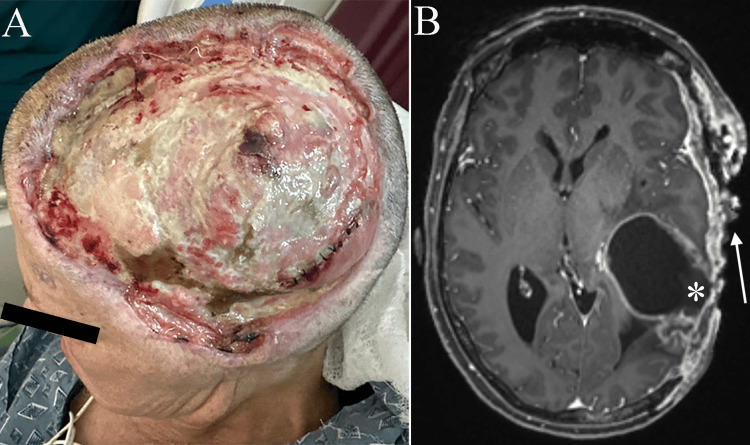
Basal cell carcinoma of the scalp with abscess (A) Pre-operative appearance of a necrotic skin defect over the left hemicranium. (B) MRI Axial T1 post-contrast gadolinium enhanced image showing skin and bone destruction with defect (arrow), dural and subdural enhancement, and left intraventricular fluid collection consistent with abscess (*).
